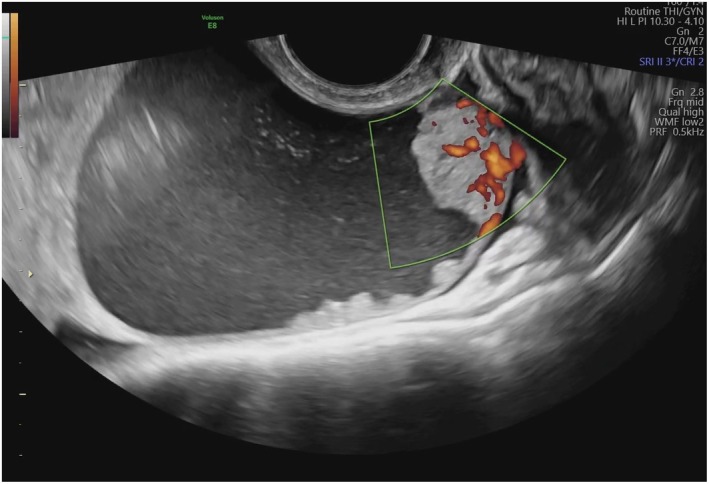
Transvaginal ultrasound image, in axial view, of a seromucinous borderline ovarian tumor, showing a left unilocular solid cyst with ground‐glass content and the presence of one papillary projection with moderate vascularization at Color Doppler (Color Score 3).
